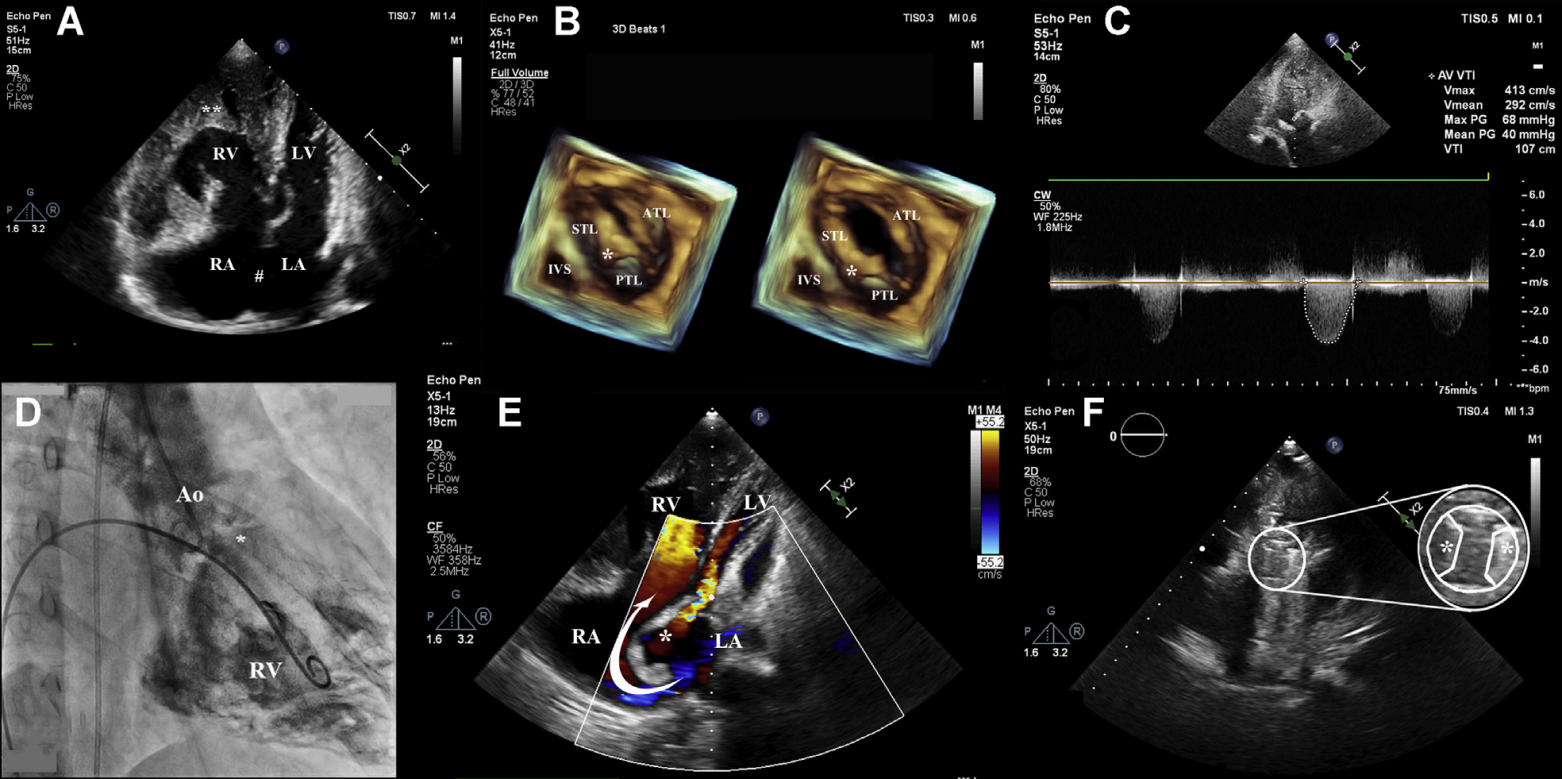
Echocardiographic and Angiographic Findings (A) Apical 4-chamber view showing a large ASD (#). RV identified to the right owing to the increased trabeculations (∗) as compared to the LV. (B) 3D Zoom images of the tricuspid valve showing thickening and fusion of leaflets with raphe between them (∗). (C) Doppler imaging of aortic valve showing severe AS. (D) Angiogram showing aorta arising from the RV and thickened aortic valve (∗). (E) Postoperative color Doppler images showing the baffle (∗) and flow of blood from the LA to the RV (arrow). (F) Demonstration of adequate disc excursion of the bileaflet (∗) prosthetic aortic valve. Also see Video 1. Ao = aorta; ASD = atrial septal defect; ATL = anterior tricuspid leaflet; IVC = inferior vena cava; IVS = interventricular septum; LA = left atrium; LPA = left pulmonary artery; LV = left ventricle; MPA = main pulmonary artery; PTL = posterior tricuspid leaflet; RA = right atrium; RPA = right pulmonary artery; RV = right ventricle; STL = septal tricuspid leaflet; SVC = superior vena cava.
